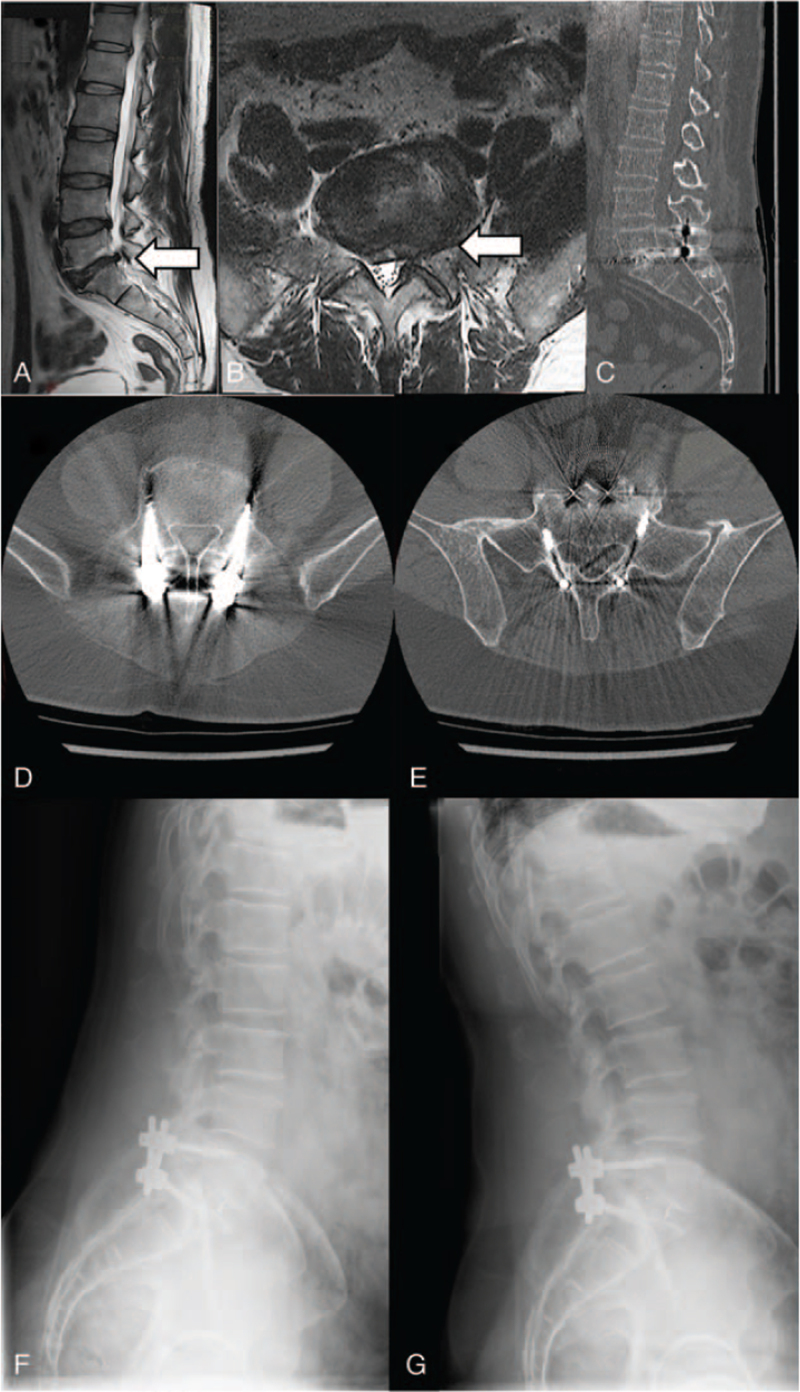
Radiological images of a patient with L5 to S1 central and critical left foraminal stenosis, which presented as left leg radiculopathy. (A) Sagittal T2-weighted magnetic resonance image of the lumbar spine shows a herniated disc at the L5 to S1 level (white arrow). (B) Axial T2-weighted magnetic resonance image shows central and left-sided critical foraminal stenosis at the L5 to S1 level (white arrow). (C) Follow-up sagittal computed tomography scan shows solid fusion status. (D, E) Follow-up axial computed tomography scans demonstrate the correct trajectory of the screws at the (D) L5 and (E) S1 levels. (F), (G) Follow-up lateral dynamic X-rays show no motion at the operated spinal level.
